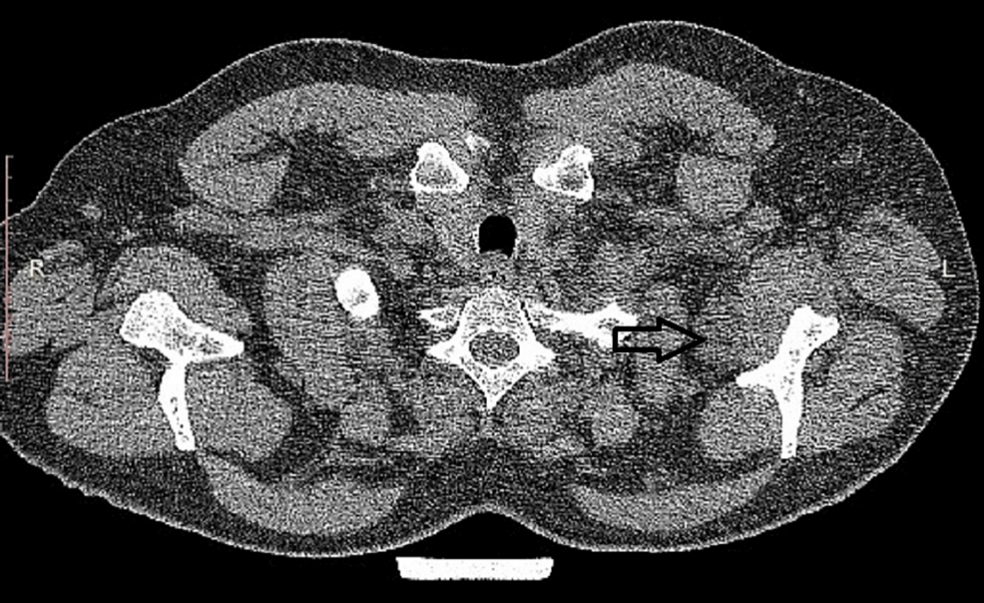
Final image from pre-operative CT showing the ganglion cyst as it extends almost 5 cm downwards into the axillary fossa
